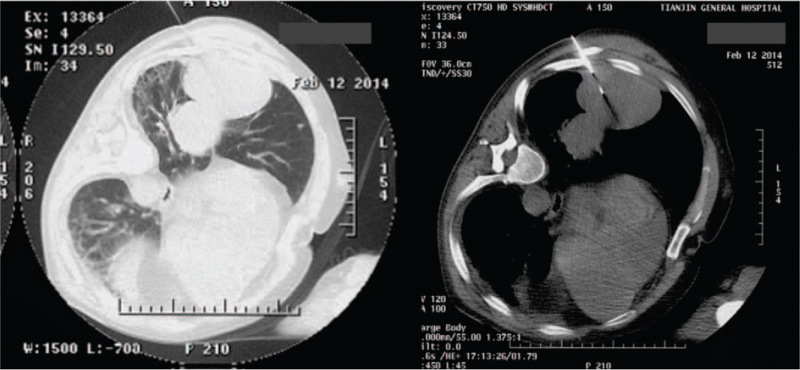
Lung lesion biopsy was performed under chest CT guidance. CT = computed tomography.
